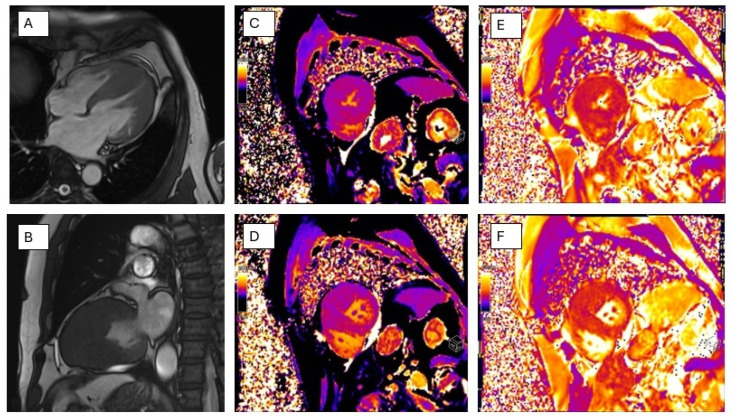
Findings in a patient with biventricular HCM. Panels (A,B) (cine CMR views): Severe asymmetric left ventricular hypertrophy with a mid-apical distribution, associated with preserved ventricular volumes and systolic function. Concomitant mid-apical right ventricular hypertrophy is observed, with preserved right ventricular volumes and systolic performance. Panels (C,D): Increased native T1 values, consistent with diffuse myocardial tissue abnormalities. Panels (E,F): Myocardial signal hyperintensity on T2-weighted images involving the mid IVS. T2 mapping demonstrates elevated T2 values at the level of the anterior wall and the interventricular septum, predominantly in the basal segments, as well as in the mid and apical inferior septum, suggestive of myocardial edema/inflammatory involvement.
